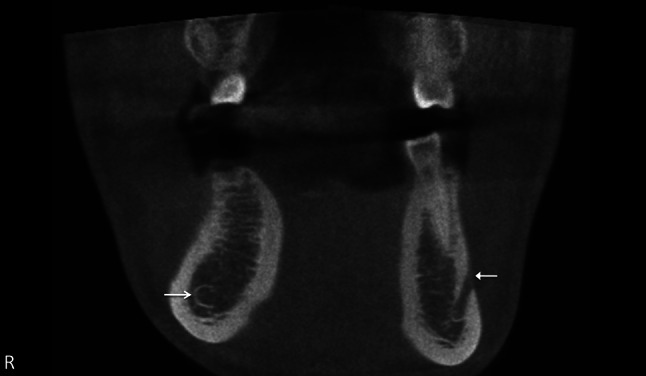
Coronal CBCT slice at level of premolars, taken for pre-implant planning on the *right side*, showing the IANC (*right side*, *arrow*) and mental foramen (*left side*, *arrow*)
